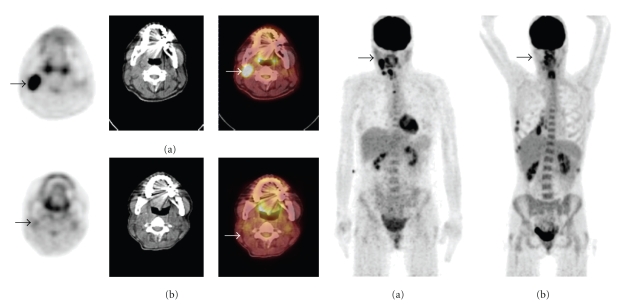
40-year-old women with right side larynx squamous cell carcinoma and a right side cervical lymph node metastases underwent PET/CT imaging before and 1 month after completion of chemoradiotherapy. (a) Pretherapy axial PET, CT, PET/CT, and MIP images reveal intense FDG uptake in the right cervical lymph node (arrows). (b) After treatment axial PET, CT, PET/CT, and MIP images reveal decrease FDG uptake in the corresponding locations (arrows). Appearance was consistent with early response to chemoradiotherapy. FDG PET/CT was valuable in monitoring early response to treatment.
